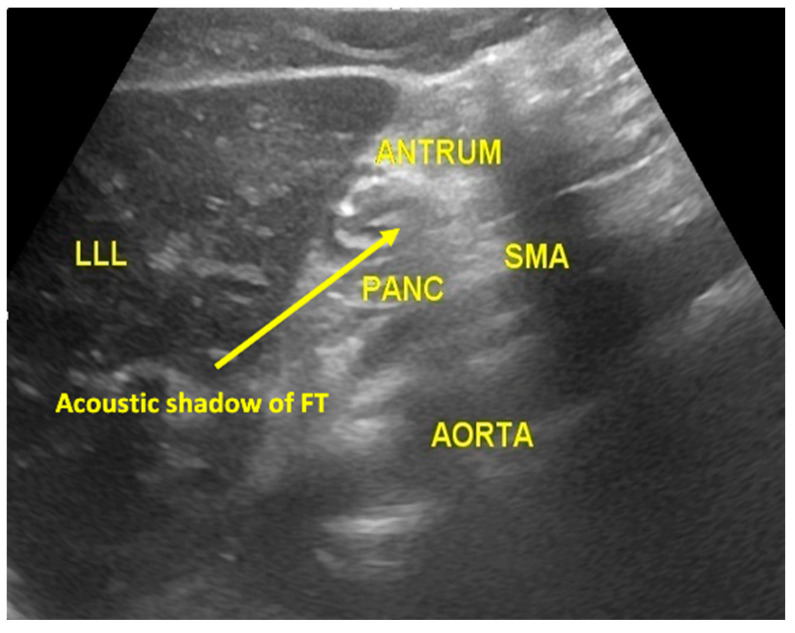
Subxiphoid area ultrasonography image (LLL = left liver lobe, SMA = small mesenteric artery, PANC = pancreas, ANTRUM = antrum of the stomach, AORTA = abdominal aorta).
